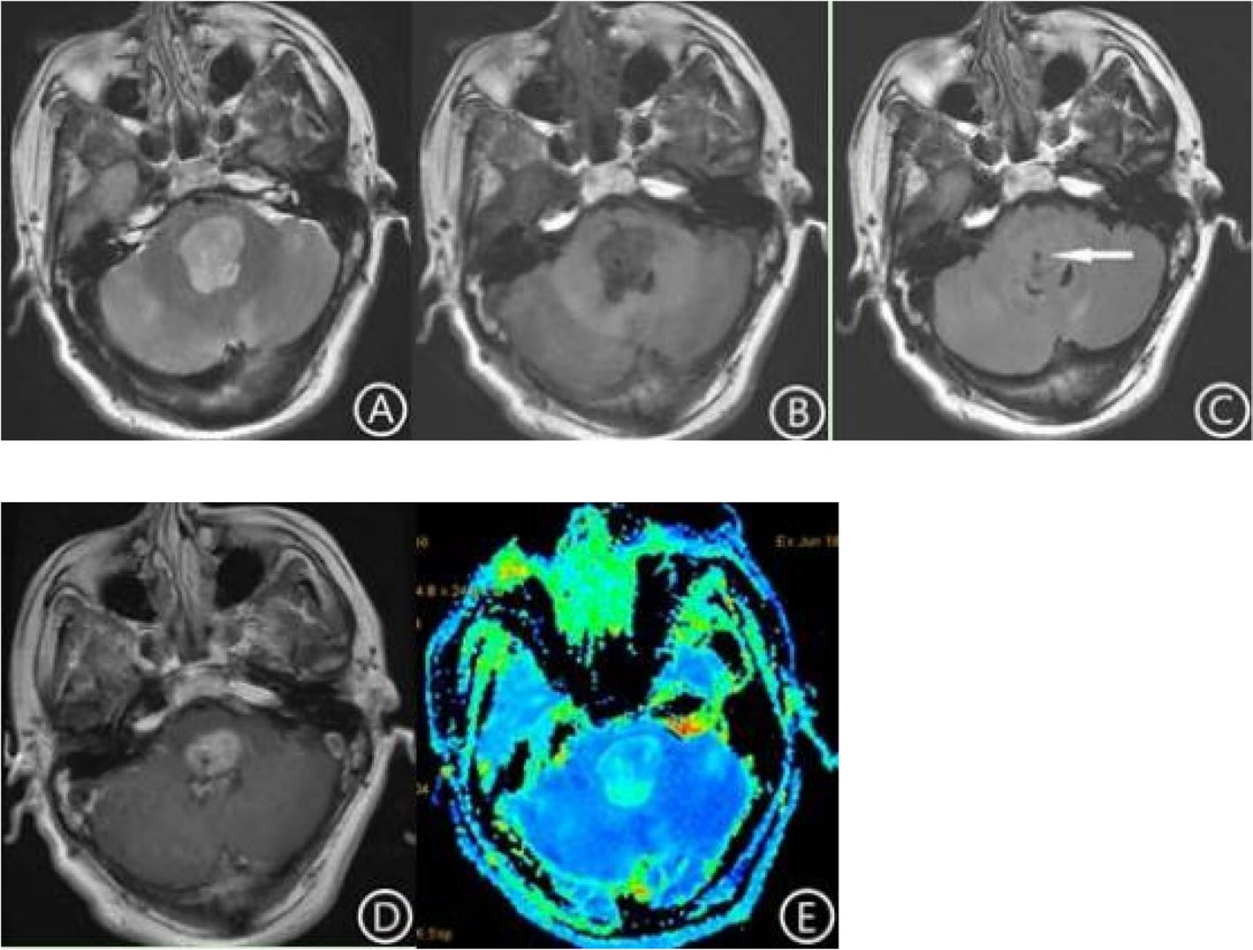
Brain MR images and ADC mapping in patients with glioma in the midline without H3 K27-altered. The tumor was located at the back of the pons and protruded into the fourth ventricle. The internal signal was uneven, T2WI showed a high signal (A), T1WI showed a low signal (B), and FLAIR showed an equal signal and cystic necrosis (C, arrow). There was no edema around the lesion. After enhancement, the tumor showed inhomogeneous and obvious enhancement (D). On ADC mapping, the lesion showed a high signal (E). The ADC value of the tumor tissue (9.65 × 10^− 4^ mm^2^/s) was higher than that of the surrounding normal brain tissue (8.95 × 10^− 4^ mm^2^/s)
